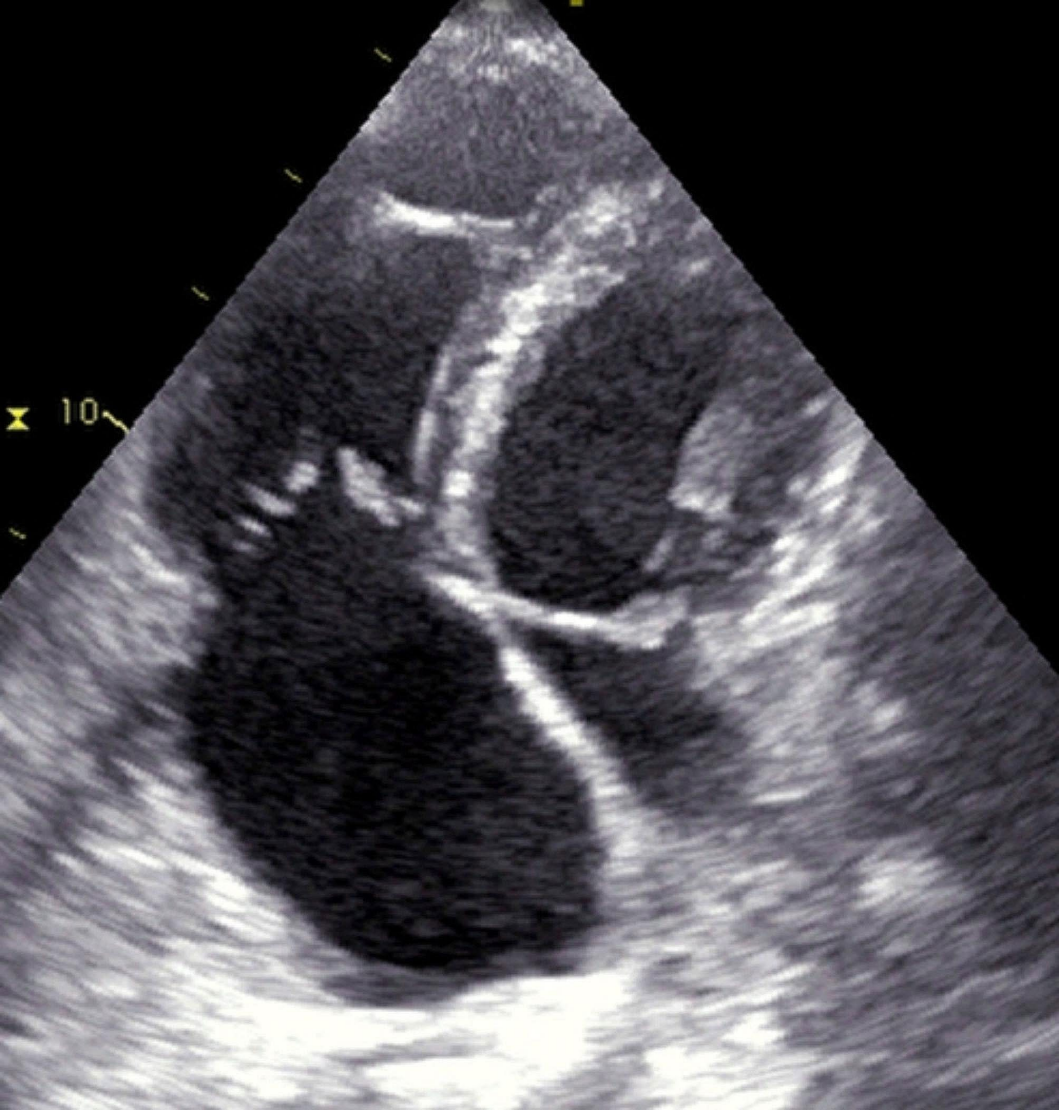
Postoperative TTE showing reconstructed tricuspid valve (modified apical four chamber view)
